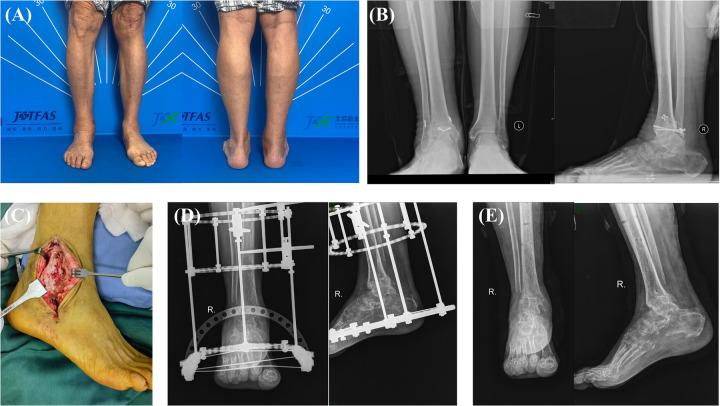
A 77-year-old male patient presented with chronic hindfoot infections subsequent to surgical intervention for right ankle fractures. The patient reported persistent pain and swelling in the right ankle, with acceptable hindfoot alignment (A). Preoperative weight-bearing x-ray imaging revealed joint destruction in the right ankle (B). Intraoperatively, purulent accumulation was noted within the right ankle joint (C). Postoperatively, x-ray evaluation demonstrated favorable fusion positioning and proper hindfoot alignment (D). At the three-month follow-up, satisfactory osseous consolidation was observed at the fusion site subsequent to the removal of external fixators (E).
